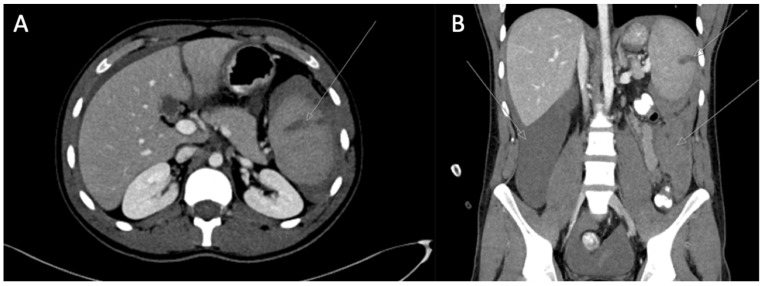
Abdomen CT scan. (A) Axial view; (B) coronal view. Arrows point at grade III spleen rupture and hemoperitoneum.
